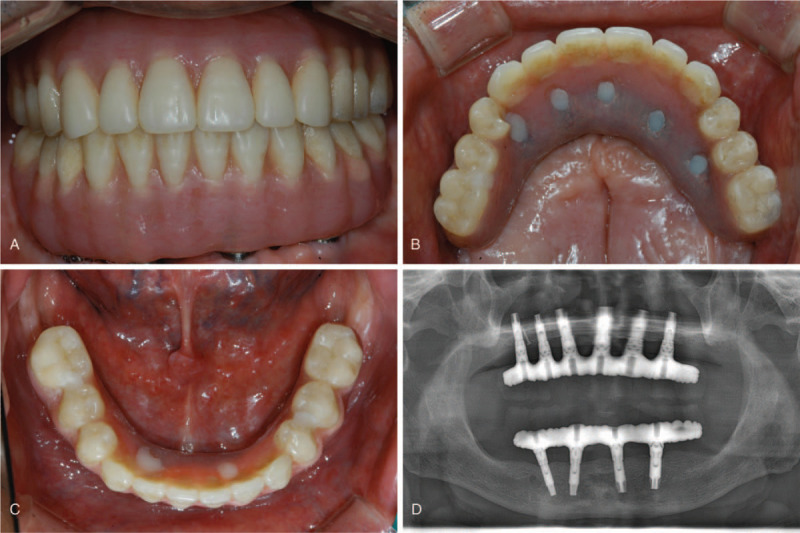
Definitive dental prosthesis. (A) Frontal view. (B) Occlusal view of the maxilla. (C) Occlusal view of mandible. (D) Panoramic radiograph after definitive prosthetic delivery (October 2008).
